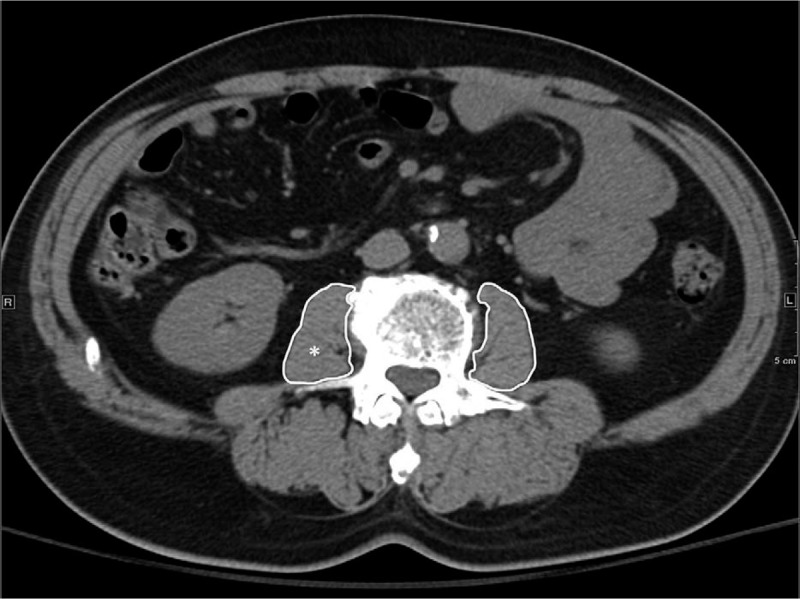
Measurement of psoas muscle area index. Psoas muscle area index [psoas muscle area (^∗^) at the mid-L3 level in CT (cm^2^)/height (m^2^)] was calculated by computed tomography within 3 months before RFA treatment with the manual trace method.
